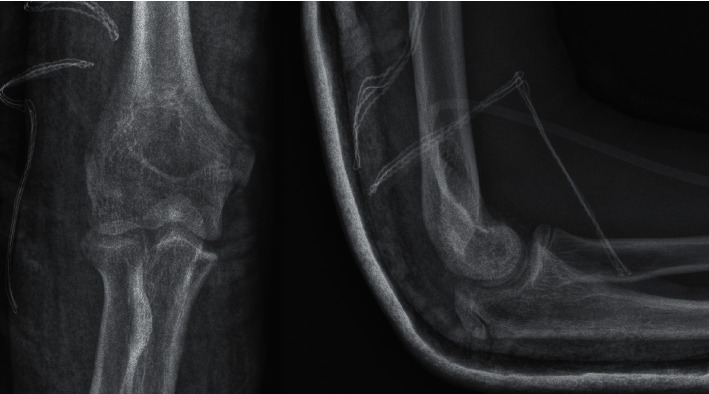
Patient number 4, postoperative X-rays. Osteochondral fragment reduced and fixated with 3 × SmartNail.
